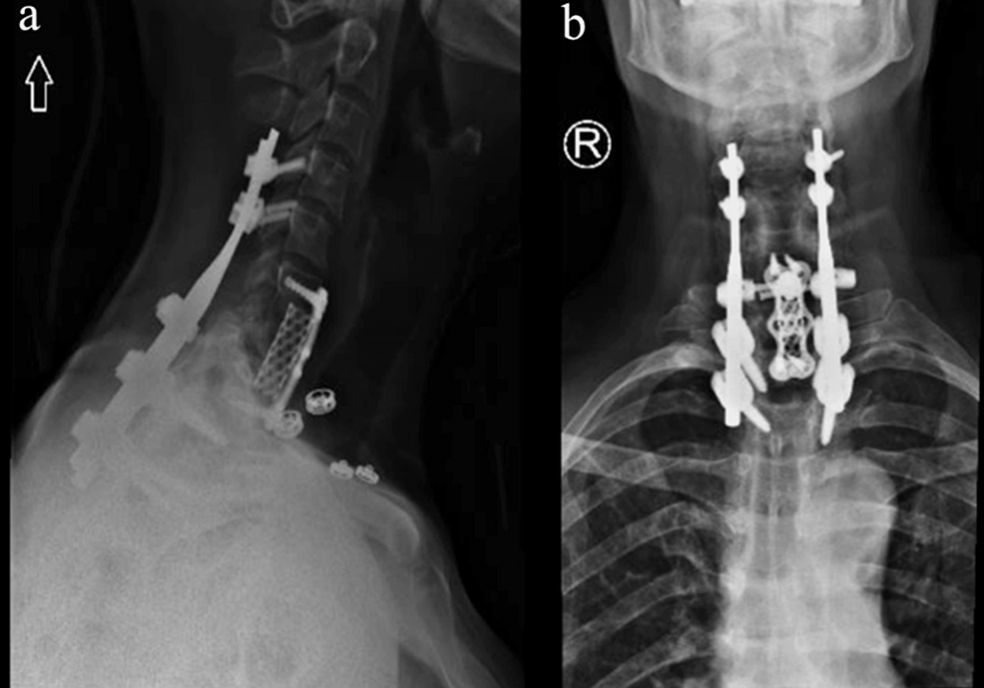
Follow-up anterior-posterior and lateral X-ray images at six months. Follow-up cervical X-ray images showing maintained reduction and alignment, and good fusion.
